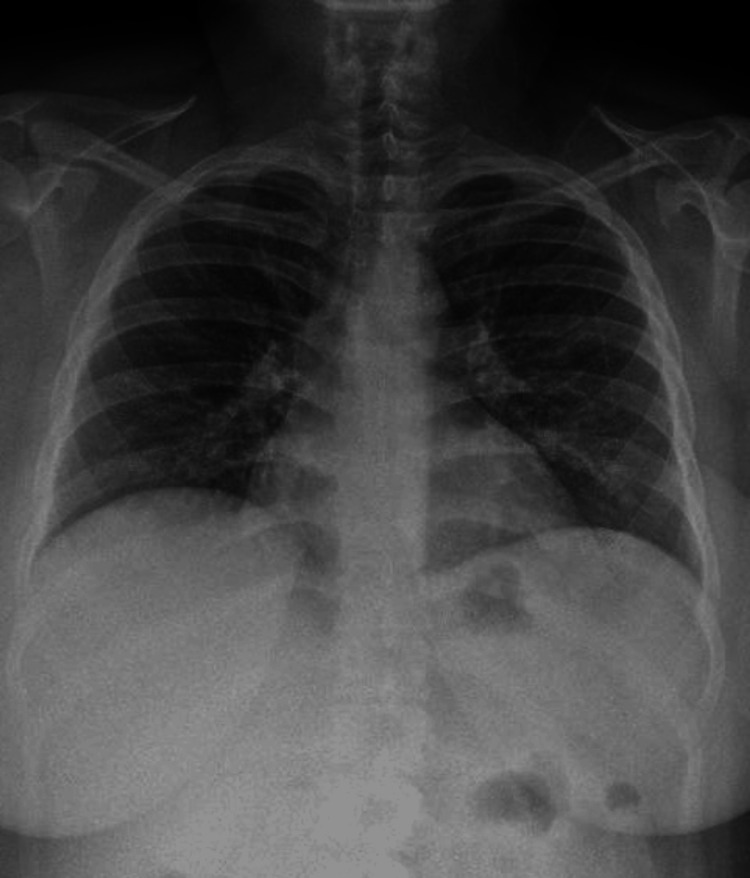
Chest X-ray, anteroposterior projection, showing the resolution of pulmonary infiltrates.
